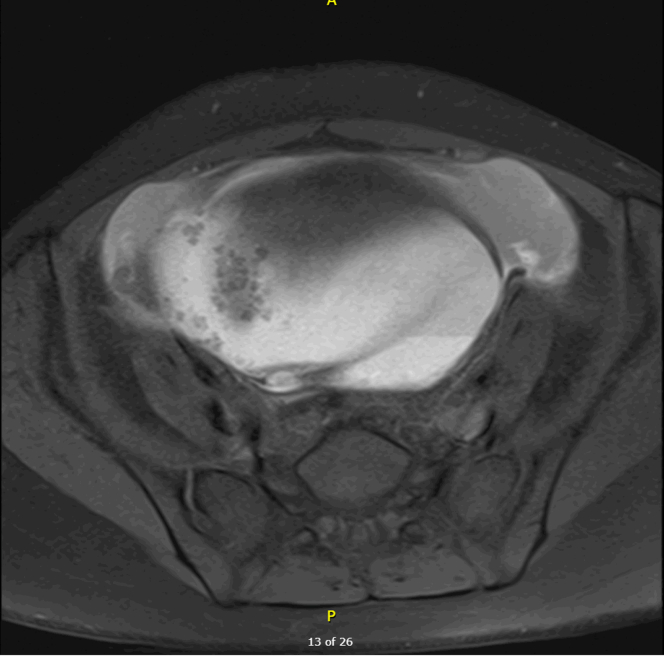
Magnetic resonance imaging of the pelvis, T1 fat suppressed, demonstrating mural nodules and endometriomas.
